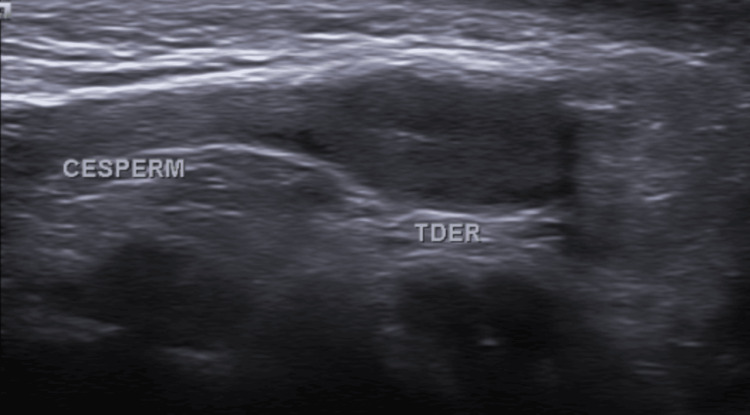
Grayscale testicular ultrasound The right testis is absent from the scrotal sac; it is identified in the inguinal region, compatible with a retractile testis
